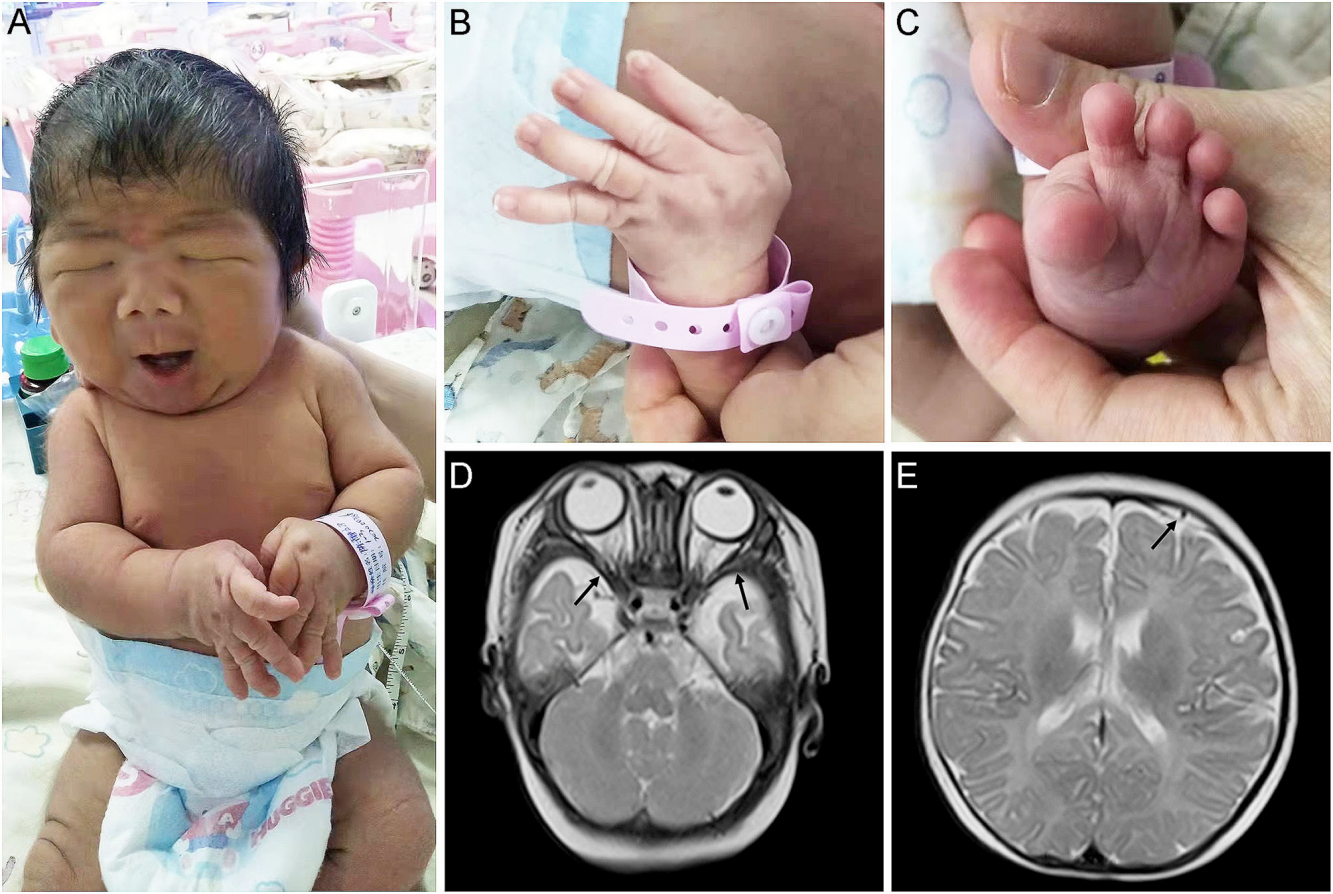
Appearance and MRI features of the proband. (A,B) Congenital contractures of the face (A) and limbs (B). (C) Deformity of the foot. (D) abnormally broadening of bilateral temporal extracranial space. (E) Subdural effusion in the left frontal area.
